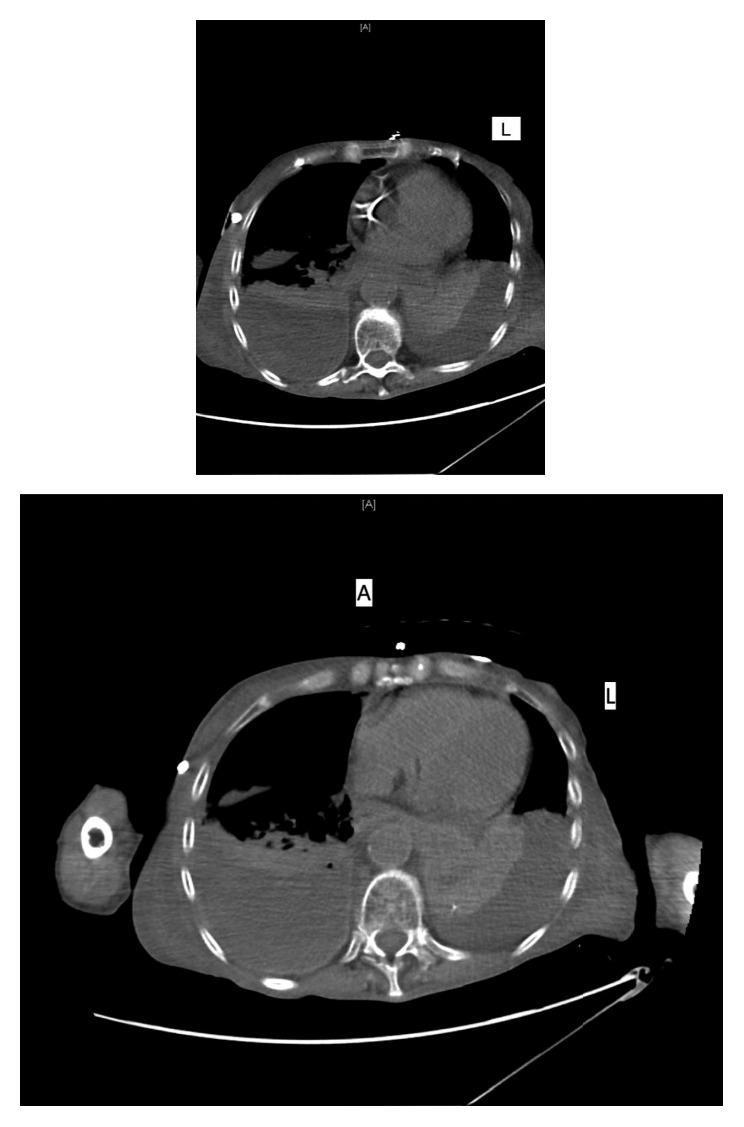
CT of the chest without contrast showing bilateral pleural fluid collections, which were larger and more complex appearing on the right.
